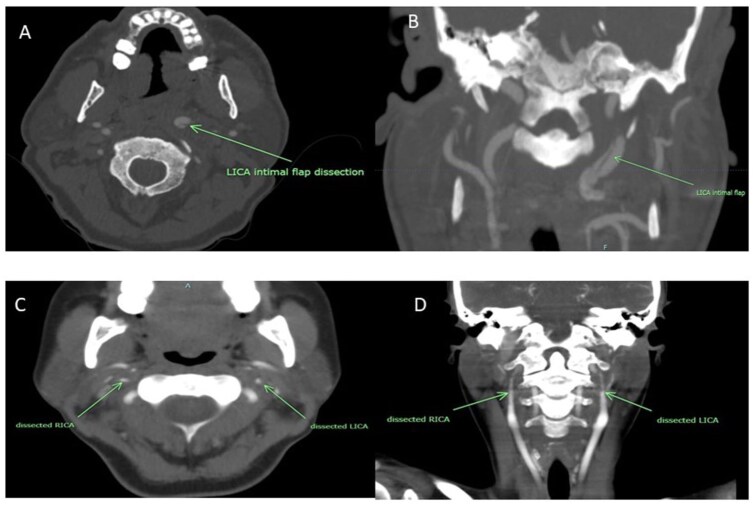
CT angiography images from patients with single (a and b) and multiple (c and d) vessel dissections in axial (a and c) and coronal (b and d) plains. Green arrows point to the dissections. LICA: left internal carotid artery; RICA: right internal carotid artery.
